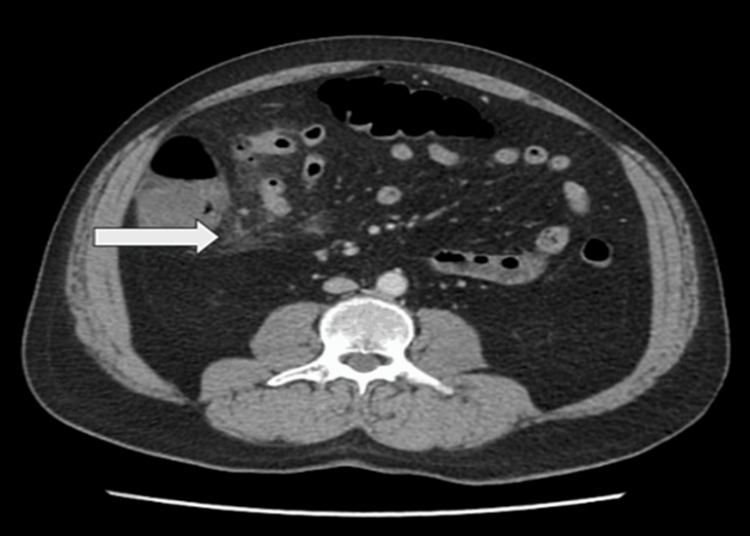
Abdominal CT scan showing fat stranding around the ileum CT: Computerized tomography
